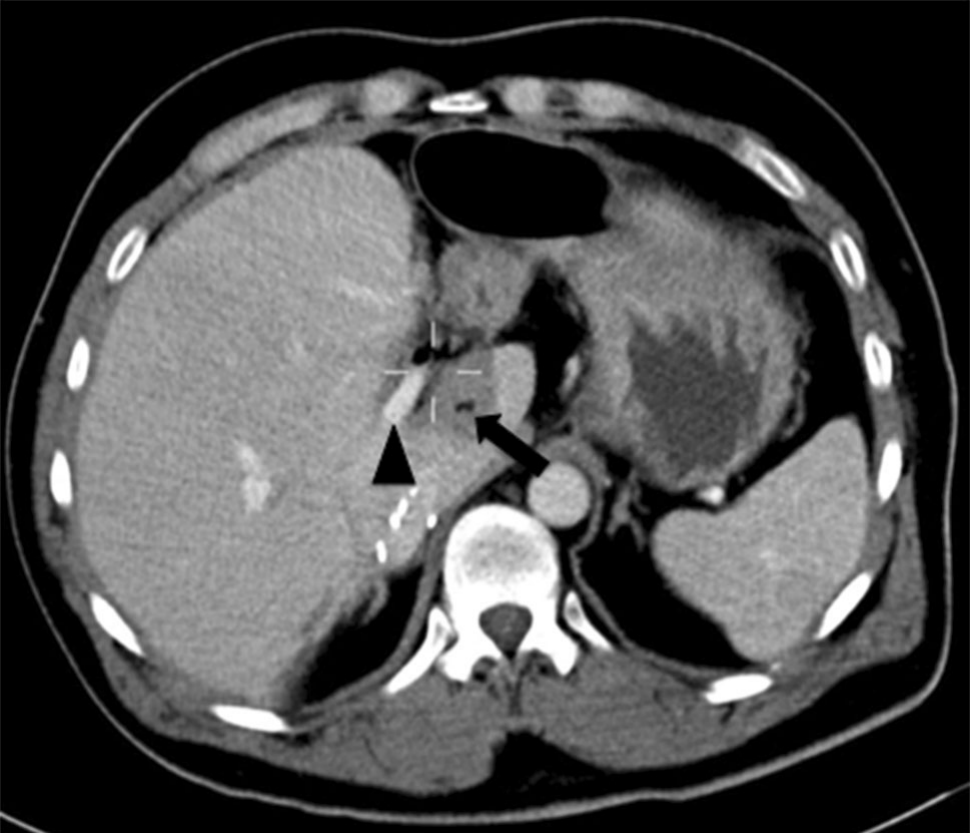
Post-treatment contrast-enhanced portal venous CT scan demonstrates the ablation zone (arrow), surrounding a portal vein branch (arrow head) which remains preserved and patent. The remains alive 8 months post-procedure
